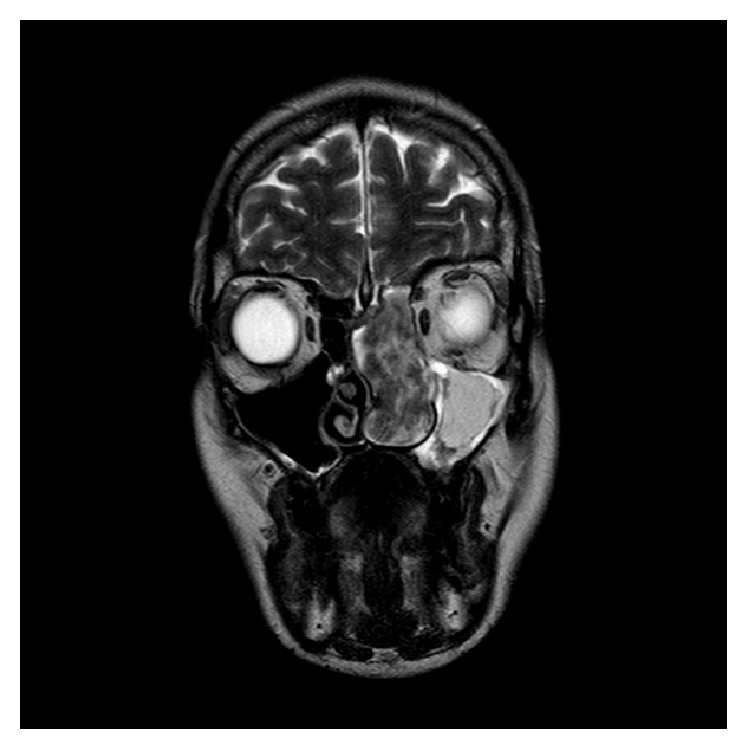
MRI (coronal, T2 weighted).
